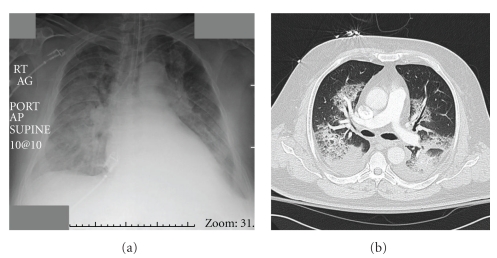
AP chest X-ray and accompanying select axial CT slice from the day of respiratory deterioration. The typical “ground glass” appearance and consolidation is noted.
